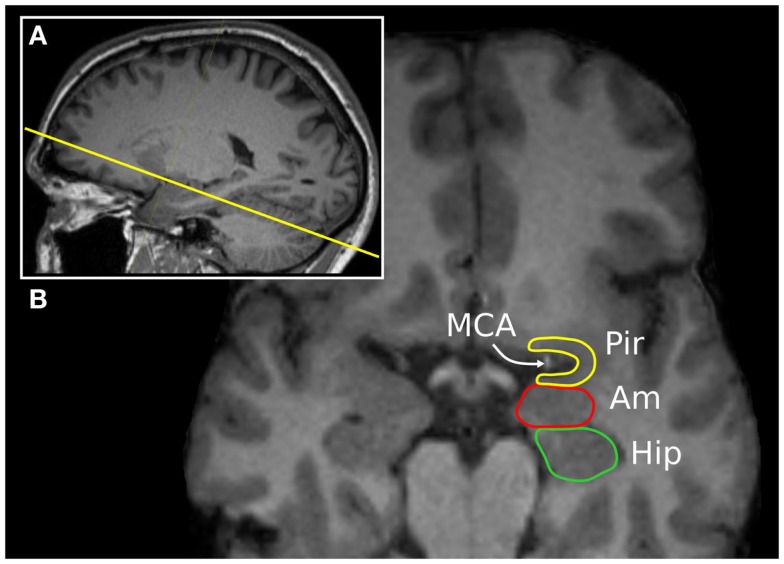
The “piriform axis”. T1-weighted MPRAGE image of a 37-year-old man, displayed in a (A) para-sagittal and (B) oblique-axial orientation, approximately +20° relative to the anterior commissure-posterior commissure axis. This orientation allows the relationship between the piriform cortex (Pir), amygdala (Am), and hippocampus (Hip) to be seen. The arrow indicates the position of the middle cerebral artery within the endorhinal sulcus.
